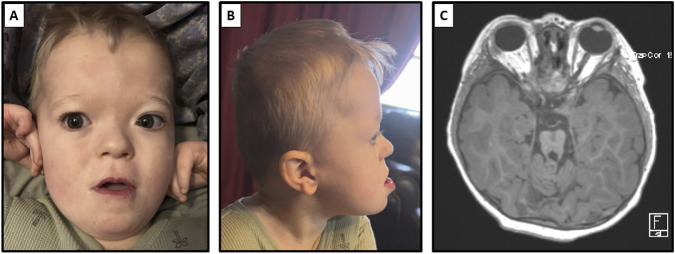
Clinical and neuroimaging features. (A,B) Frontal and profile views of the patient demonstrating microcephaly, brachycephaly, occipital flattening, midface flattening, hypertelorism, sparse medial eyebrows, and prognathism. (C) Brain MRI showing corpus callosum thinning with dysplastic fornices, and thickening of the irregular superior cerebellar peduncles with vermian dysplasia/hypoplasia and loss of the decussation of the superior cerebellar peduncles.
